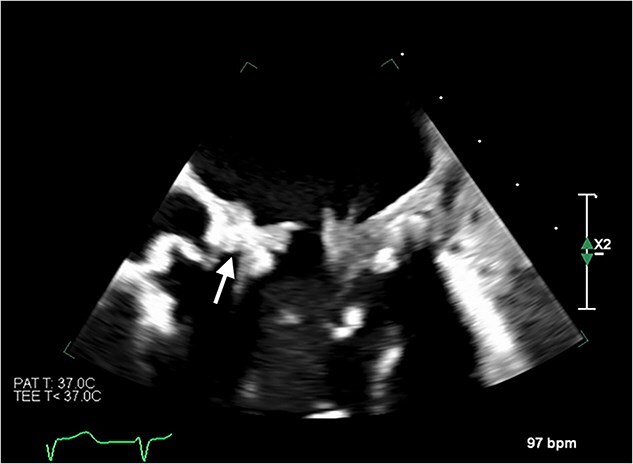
Transoesophageal echocardiogram showing thromboses on the bioprosthetic mitral valve leaflets, as well as in the left atrium and the inferior vena cava. Thrombus is also visible on the non-coronary cusp of the aortic valve.
